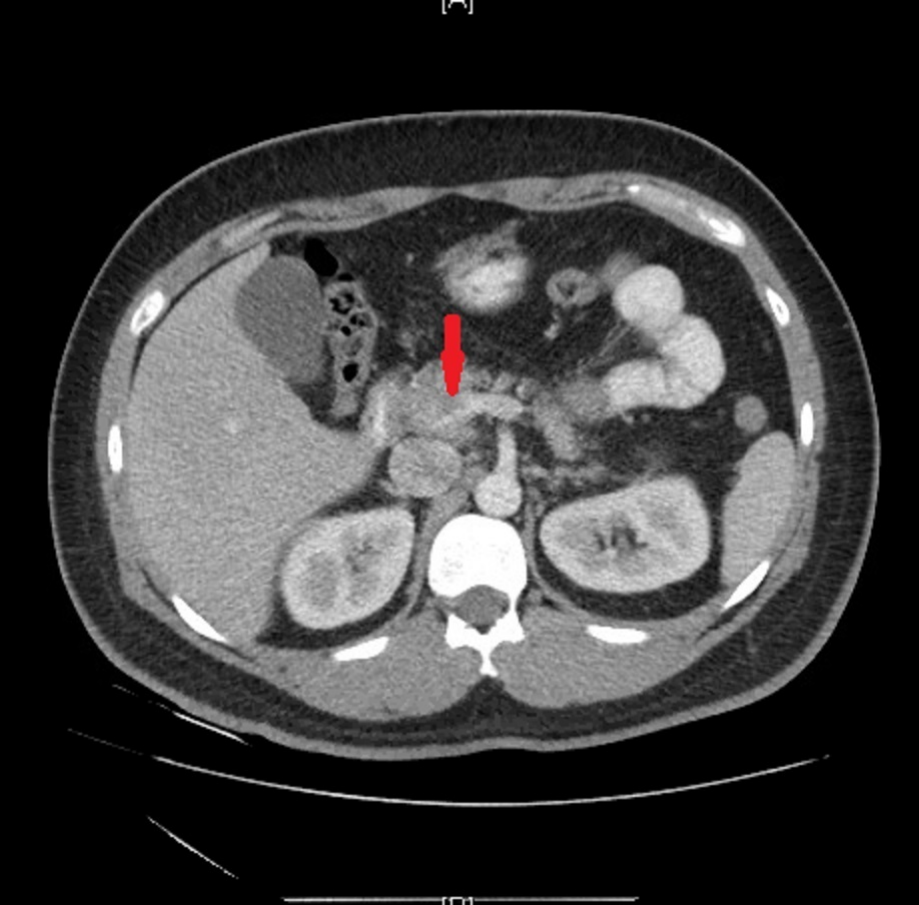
Computed tomography of the abdomen (axial view) showing portal vein thrombus
